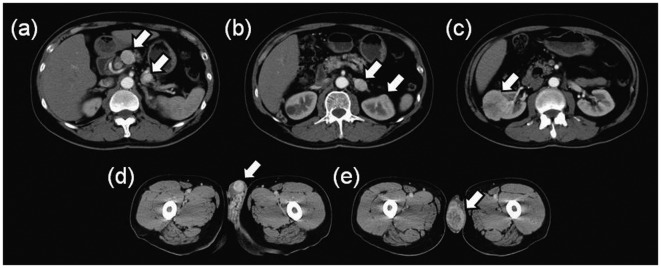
Abdominal computed tomography showing multiple tumors in various organs (arrows), including the (a) pancreas, (b) left adrenal gland and left kidney, (c) right kidney, (d) right testis and (e) left testis.
